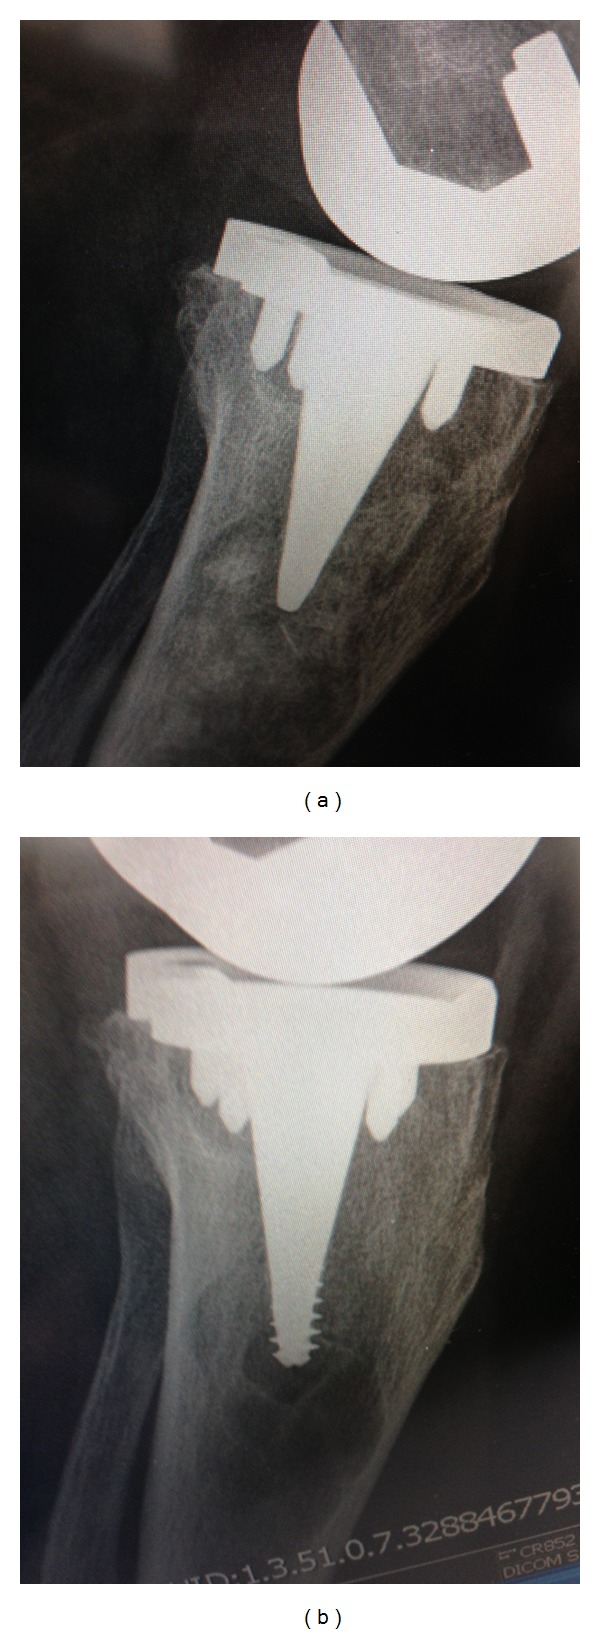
Radiographs for a 64-year-old female patient who underwent bone grafting and isolated insert exchange. (a) prerevision AP view radiograph showing osteolytic region. (b) Three-month postrevision AP view radiograph.
